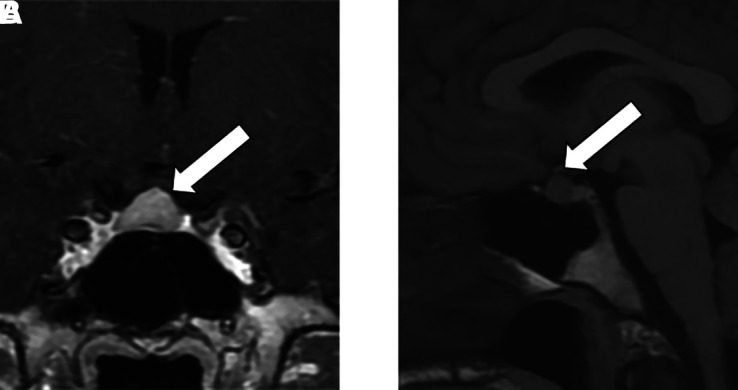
Coronal (A) and sagittal (B) views of the pituitary gland in a brain MRI. The pituitary is mildly enlarged with rounded convexity of the superior margin (white arrows).
